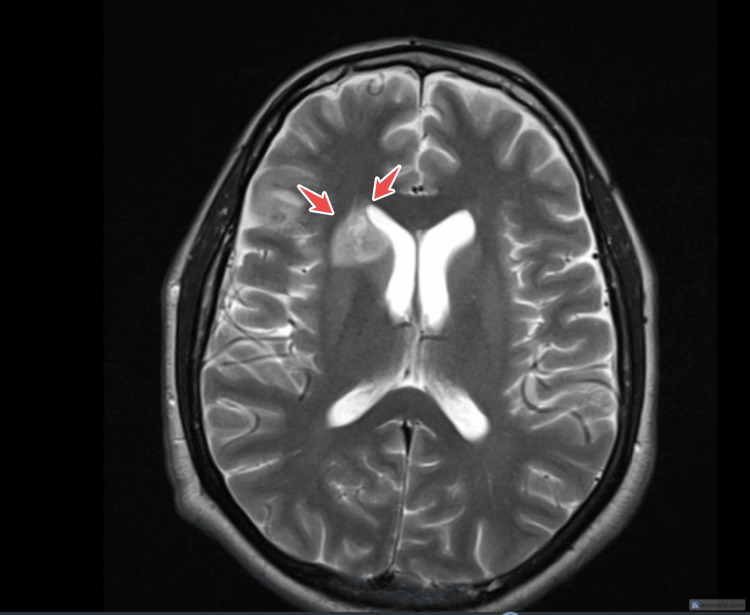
MRI brain showed ischemic stroke. MRI: Magnetic Resonance Imaging.
